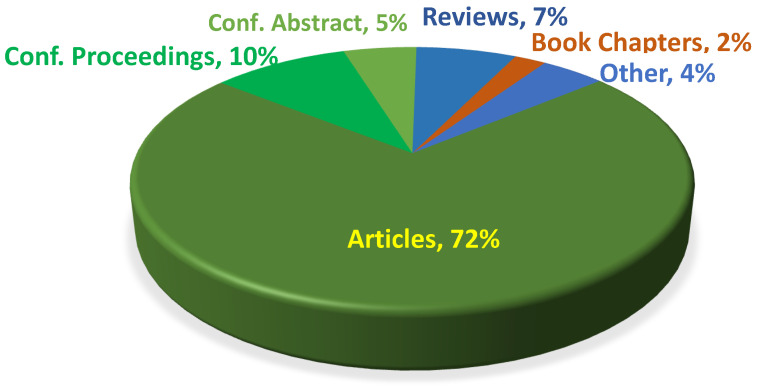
Averaged distribution of scientific papers on mechanochemistry, from searches of various combinatorial keywords: (mill/cavitation/extrusion/(sonication/ultrasonic)/ultrasound)/(mechanochemistry/mechanochemical)/(macromolecule/biomacromolecule) (last: 15 October 2023).
